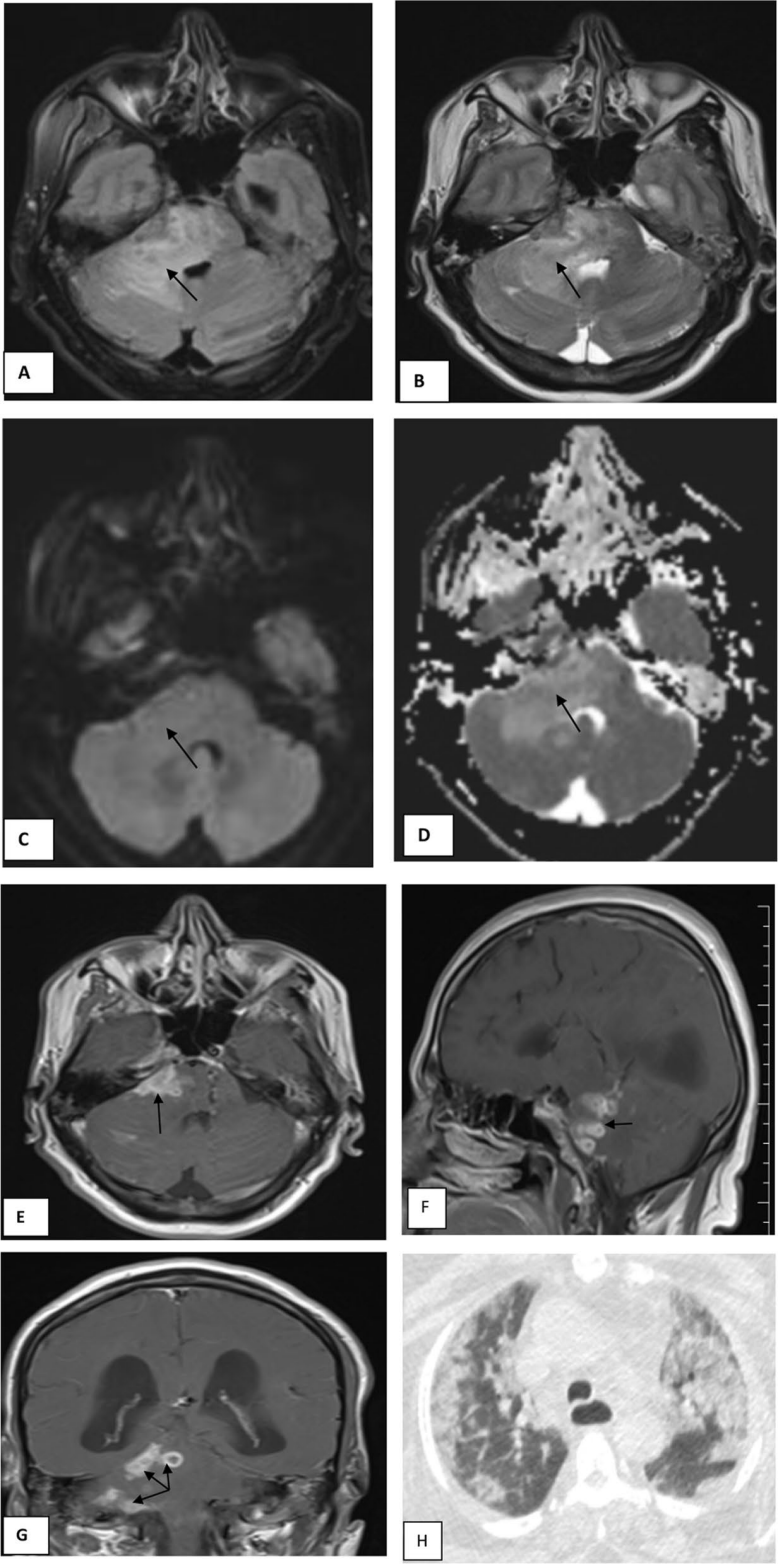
Male patient aged 48 years with recent history of COVID-19 since 3 weeks presented with fever, convulsions, altered consciousness and lethargy. FLAIR and T2WI sequences (A, B) show patchy area of high signal intensity seen at right mid brain, pons, right middle cerebellar peduncle as well as right cerebellar hemisphere. Diffusion images and ADC (C, D) show free diffusion (black arrows). Axial, sagittal and coronal (E–G) post-contrast T1 images show multiple ring enhancing lesions at fore-mentioned sites, this together with enhancing nodular meningeal thickening at right cerebello-pontine angle, right ambient cistern, right meckle’s cave and right cavernous sinus (black arrows) … this picture suggestive for post-COVID-19 meningoencephalitis. Axial CT (F) chest cut of the same patient shows bilateral peripheral ground-glass opacities mixed with crazy-paving appearance (severe severity)
